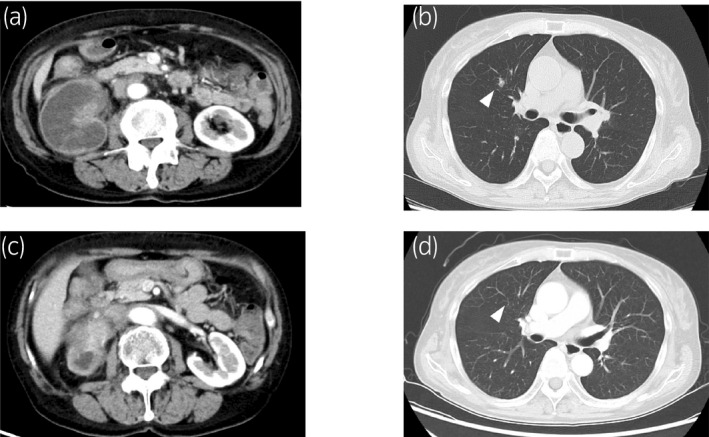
(a) CT scan shows a right renal pelvic mass and para‐aortic lymph node swelling at the time of pembrolizumab initiation. (b) CT scan shows a lung metastasis (arrowhead) at the time of pembrolizumab initiation. (c) CT scan shows a primary right renal pelvic tumor shrinkage after 6 months from pembrolizumab initiation. (d) CT scan shows disappearance of lung metastasis (arrowhead) after 6 months from pembrolizumab initiation.
